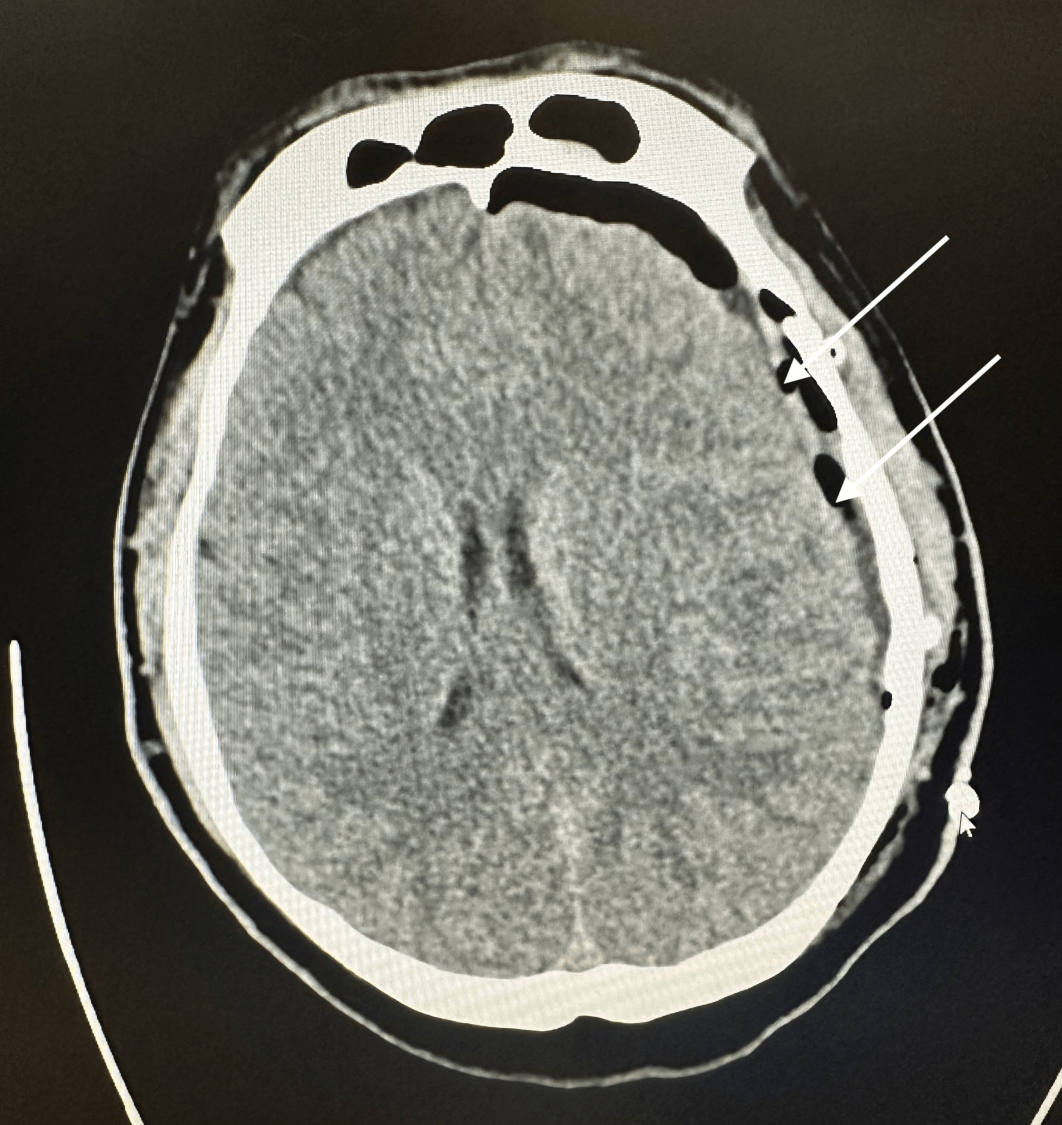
Transverse CT image post-craniotomy demonstrating resolution of SDH CT, computed tomography; SDH, subdural hematoma
